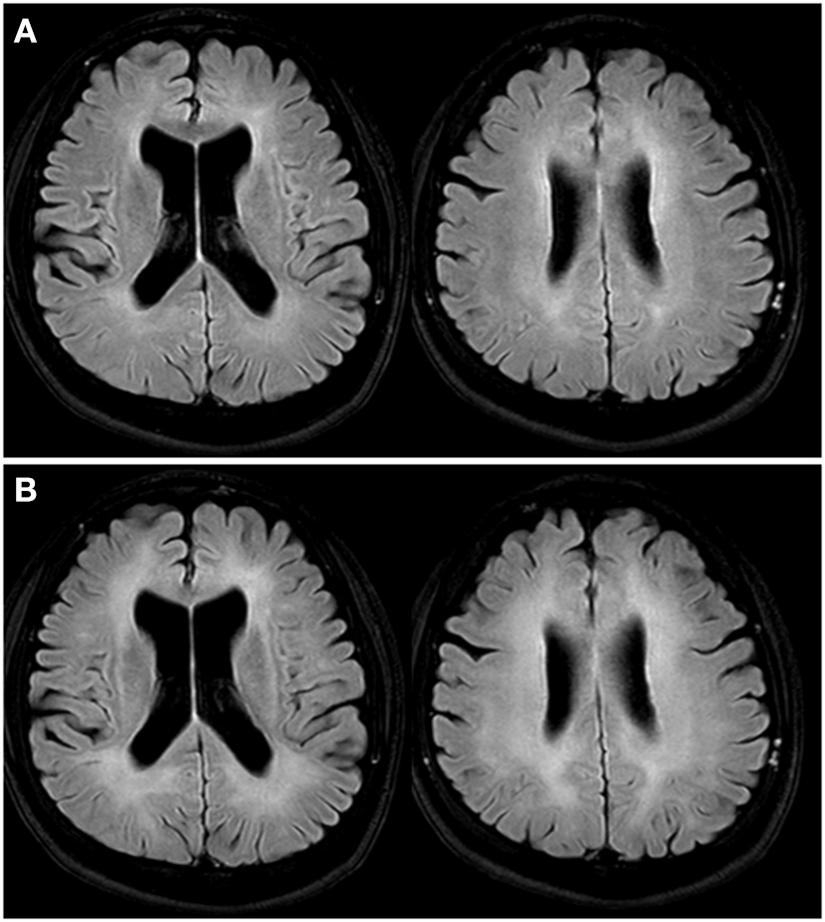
Brain MRI T2 fluid-attenuated inversion recovery image. (A) Initially, it shows bilateral symmetric confluent high signal intensities in periventricular white matters of cerebral hemispheres, which are compatible with delayed CO encephalopathy. (B) After HBOT shows increases in the extent of confluent high signal intensities in bilateral cerebral periventricular and subcortical white matter. MRI, magnetic resonance imaging; CO, carbon monoxide; HBOT, hyperbaric oxygen therapy.
